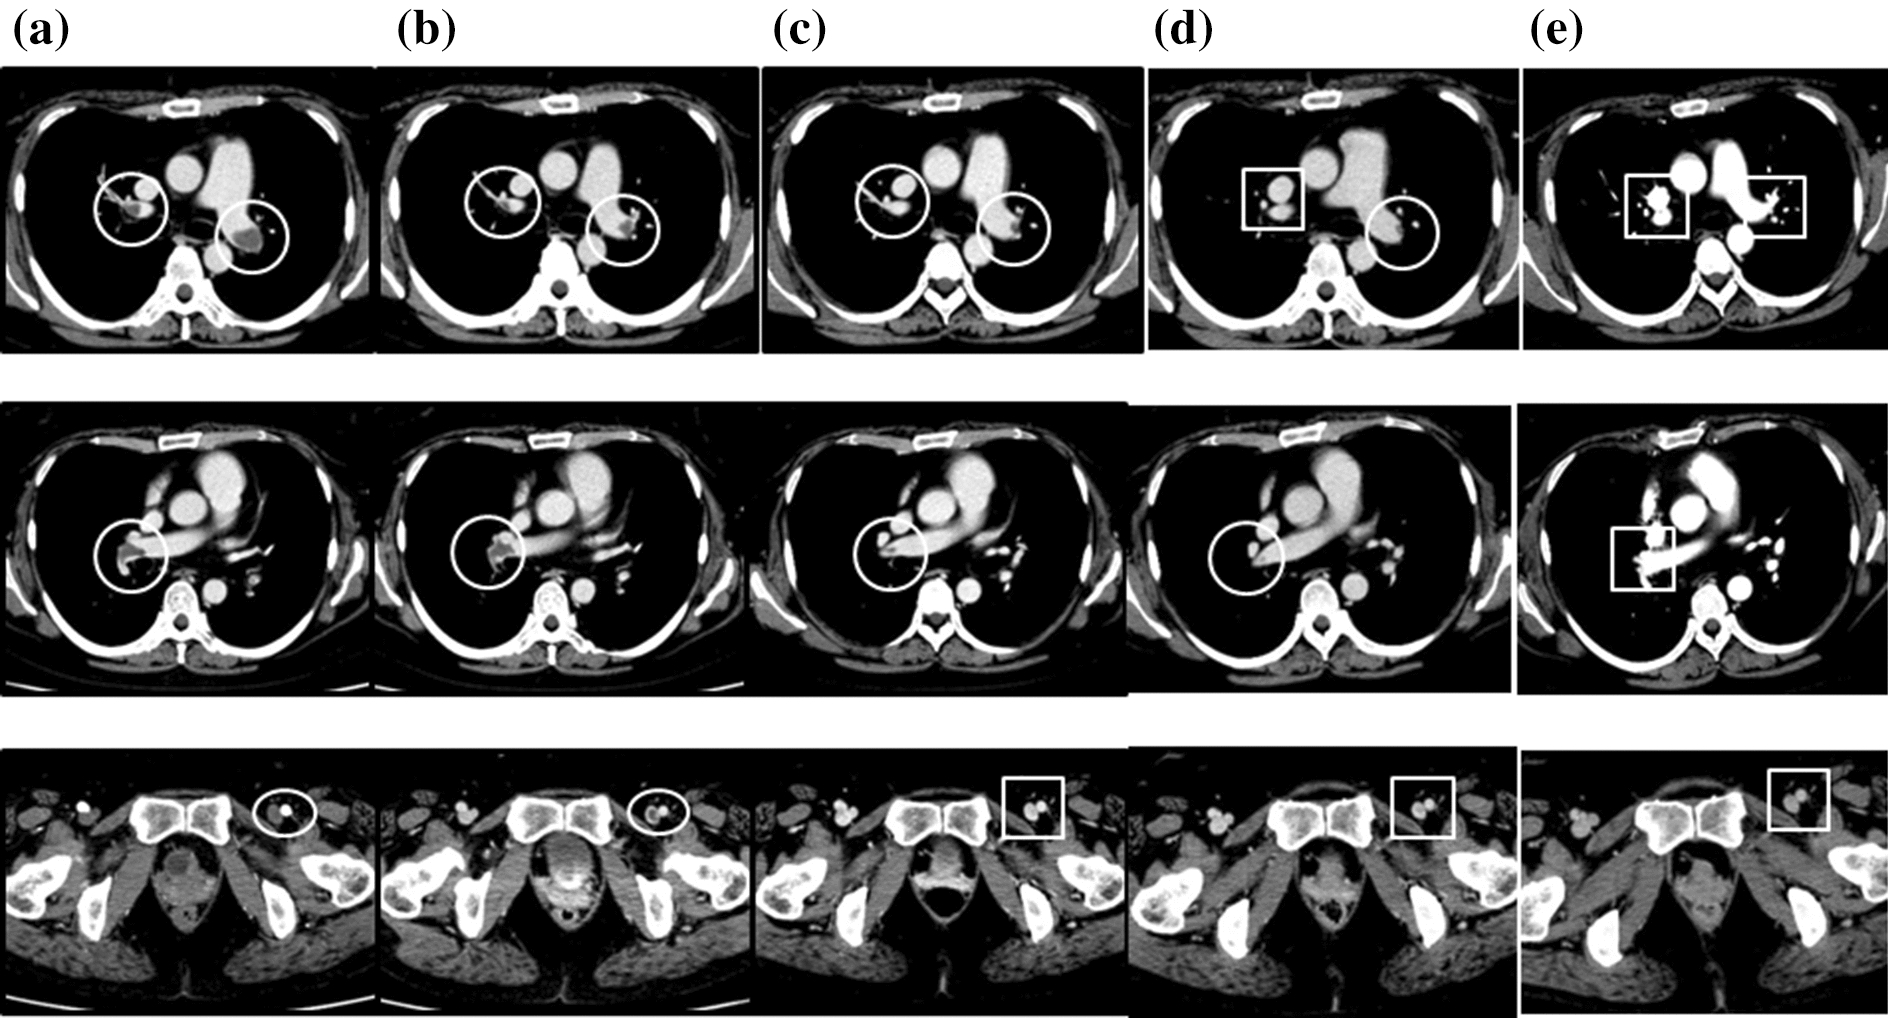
Rituximab-associated changes in the thrombi in both pulmonary arteries and the left common femoral vein thrombus on contrast-enhanced computer tomography (CT). Contrast-enhanced CT images before rituximab initiation (a), immediately after the first course of rituximab (500 mg/week for 4 weeks, repeated 6-monthly) (b), and at 3 months (c), 1 year (d), and 2 years 2 months (e) after the start of rituximab. The thrombi observed before rituximab initiation immediately started to improve with rituximab therapy. Open circles, thrombus present; open squares, thrombus has disappeared
